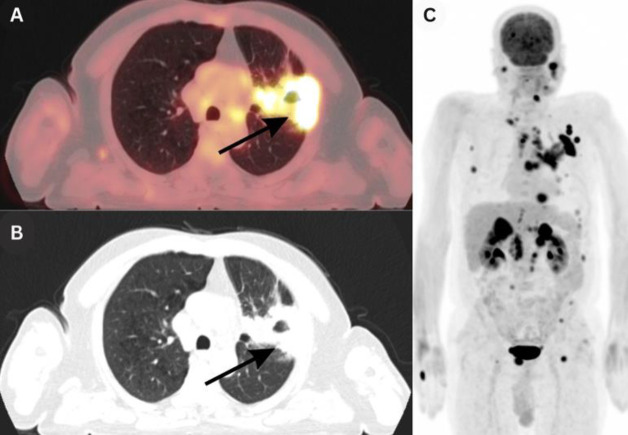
Fused (A) and CT (B) images of ^18^F-FDG PET/CT scan on axial plane, lung window showing a large spiculated soft tissue mass in the left upper lobe, with cavitary component (black arrows), this mass is associated with consolidative changes and pleural tethering, infiltrating the left 3rd and 4th ribs. The mass is exhibiting an intense FDG uptake (SUV_max_: 20.7), and measuring collectively with the consolidative part of it (5.1×7.4 cm) in maximal axial dimensions. Maximum intensity projection (MIP) demonstrating hypermetabolic widespread metastases (C)
